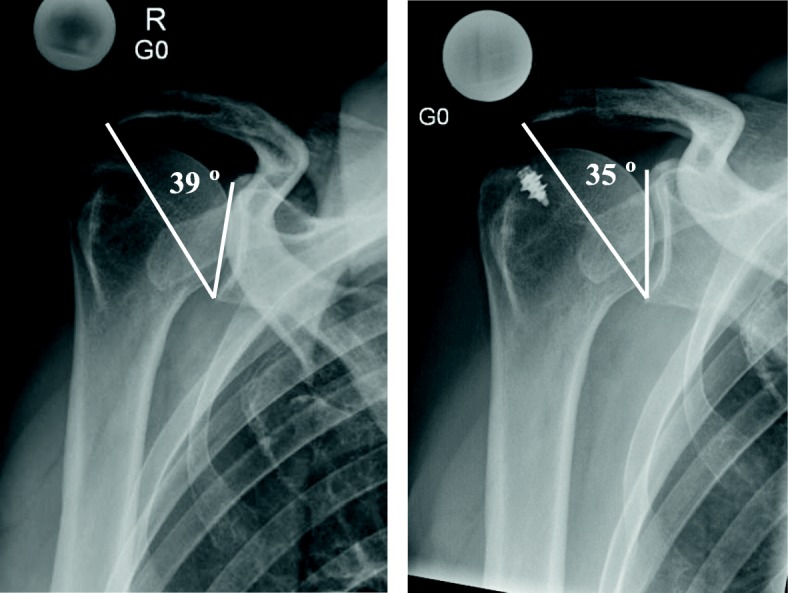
Pre- and postoperative x-ray (*p1 scapula*) reducing CSA from 39° to 35° by anterolateral acromioplasty
